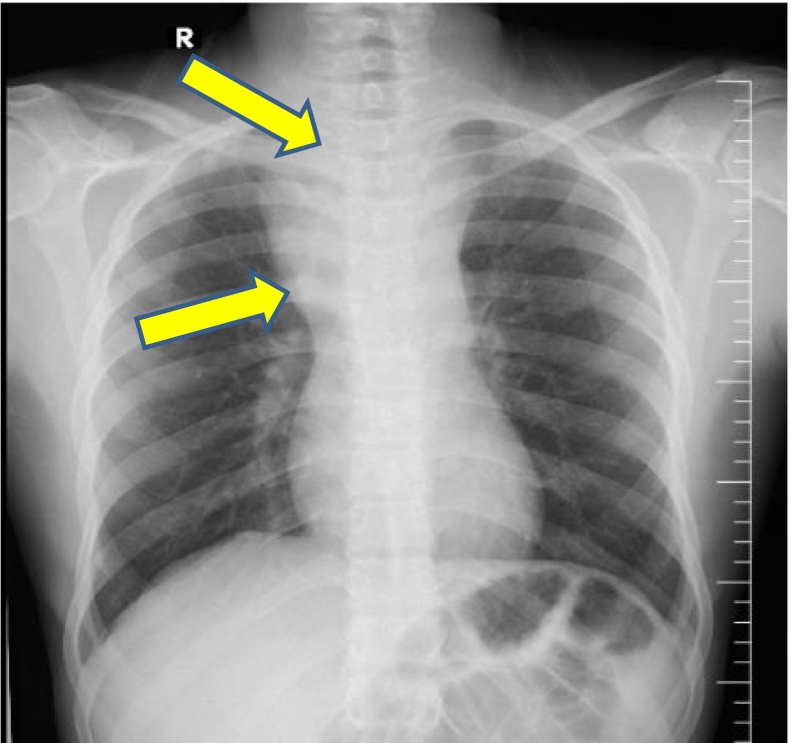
A chest x-ray showed a mediastinal mass and comminuted fracture of the sternoclavicular joint.
